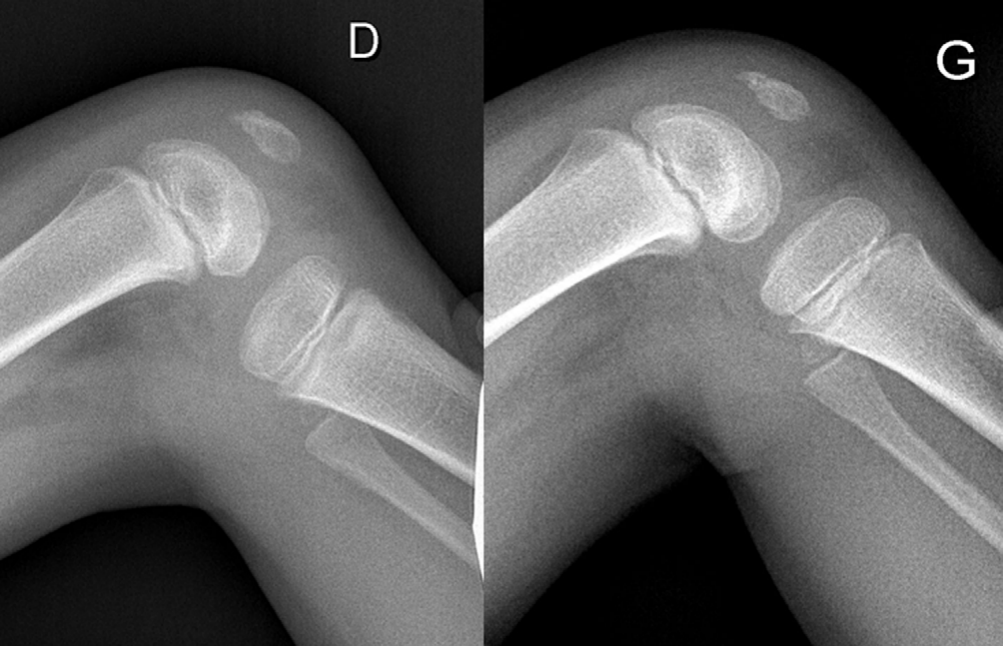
Case 1. Posterior drawer sign with more 10 mm on stress radiographs of the right knee. No bony avulsion was noticed.Figure 2 F2:
